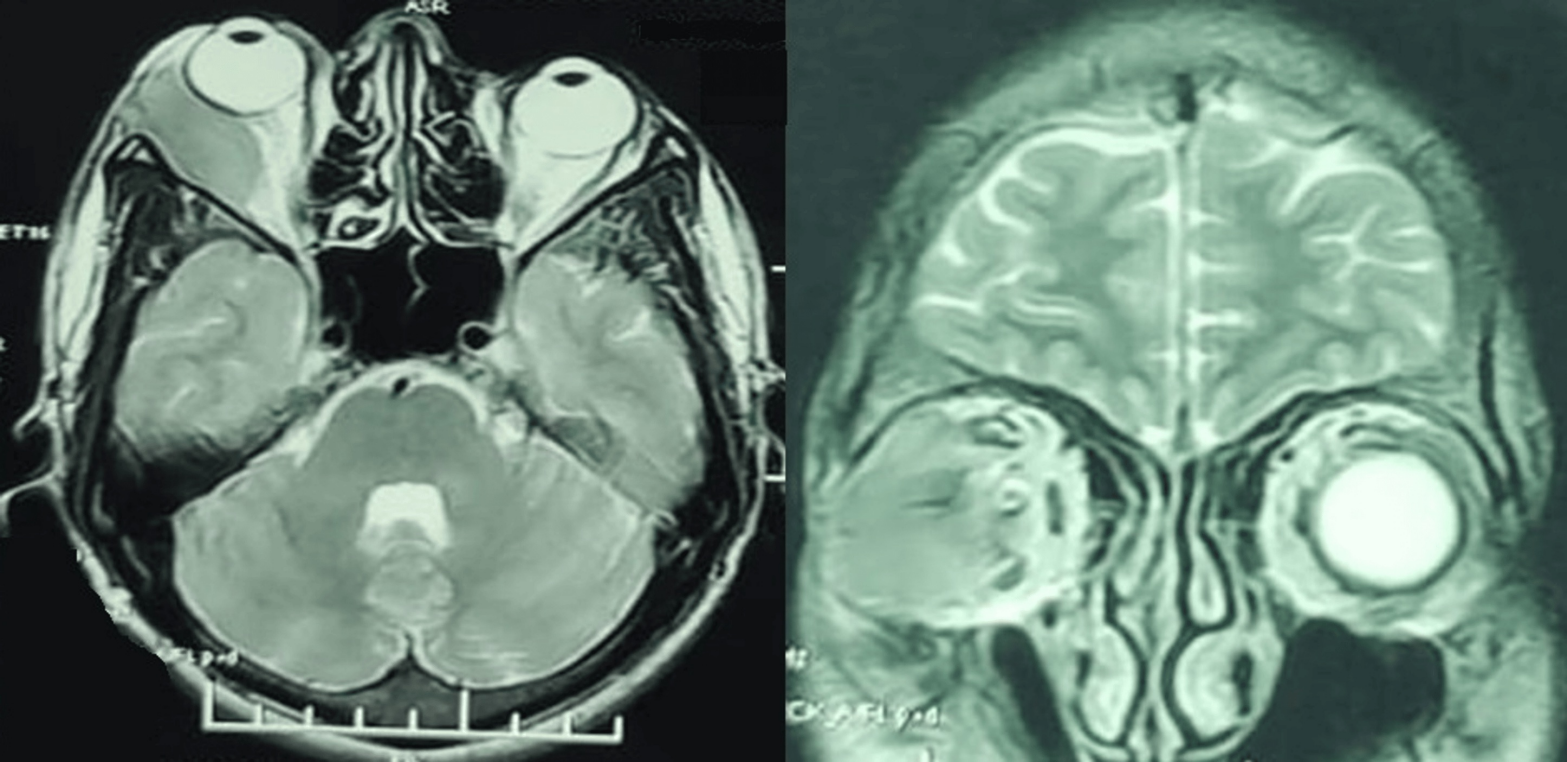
T2-weighted MRI showing isointense altered signal intensity lesion with diffusion restriction noted at lateral aspect of extraconal space of right orbit.
